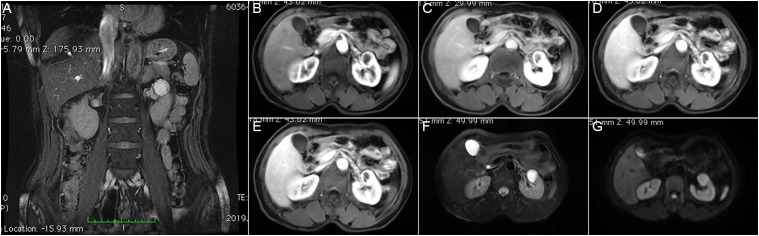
MRI characteristics of the cystic renal lesion. The lesion measured 21 × 18 mm, showing low signal intensity on T1WI, high signal intensity on T2WI and DWI, and peripheral enhancement with internal irregular non-enhanced areas on contrast-enhanced imaging. (A) Coronal T2-weighted image. (B–E) Axial contrast-enhanced images obtained at different phases. (F) Axial T2-weighted image. (G) Axial diffusion-weighted image.
